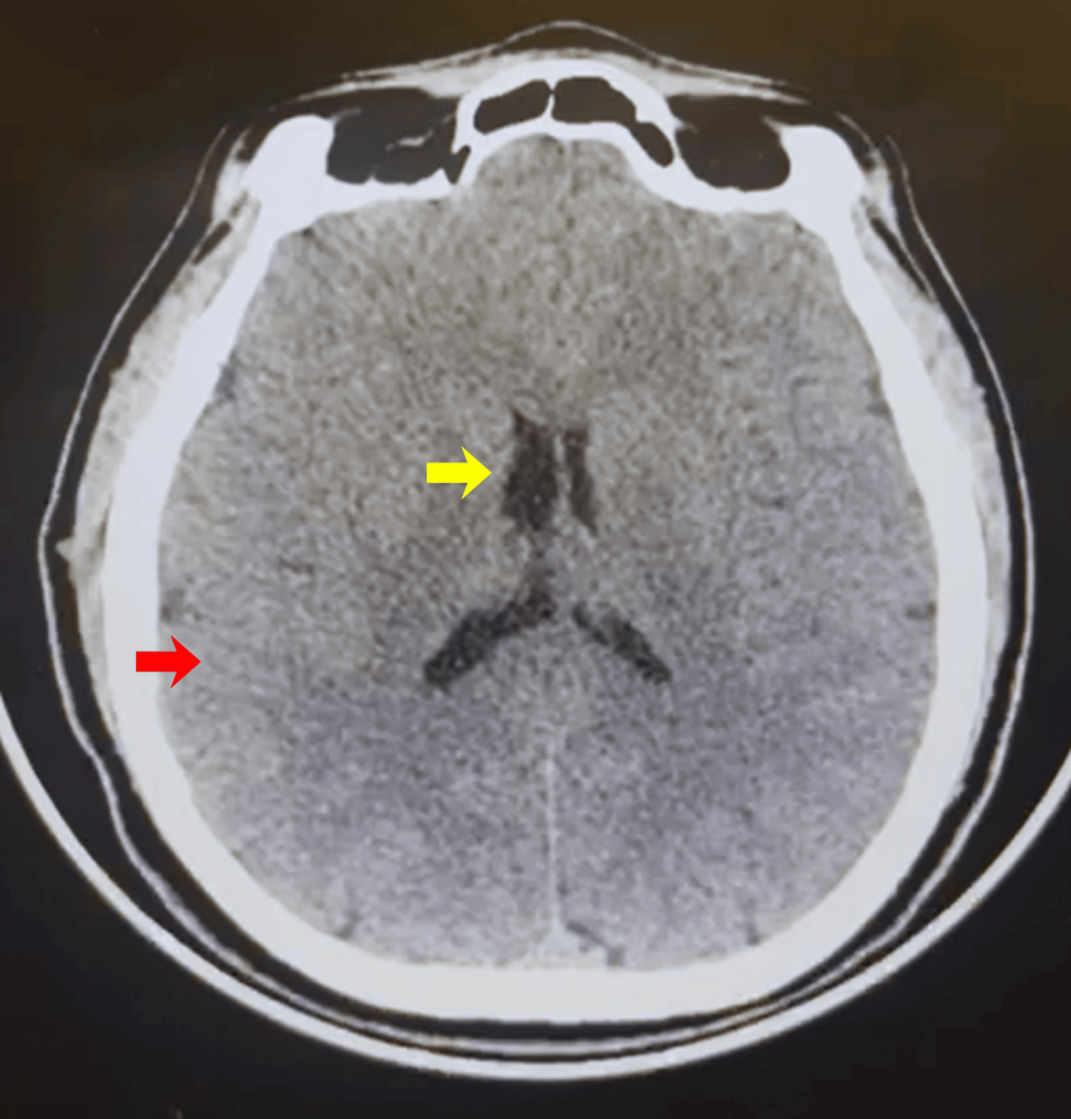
Axial non-contrast brain computed tomography. Axial non-contrast brain computed tomography demonstrating preserved gray-white matter differentiation and no evidence of intracranial hemorrhage, mass effect, or acute ischemic changes. The yellow arrow indicates the lateral ventricle, and the red arrow indicates preserved gray-white matter differentiation.
